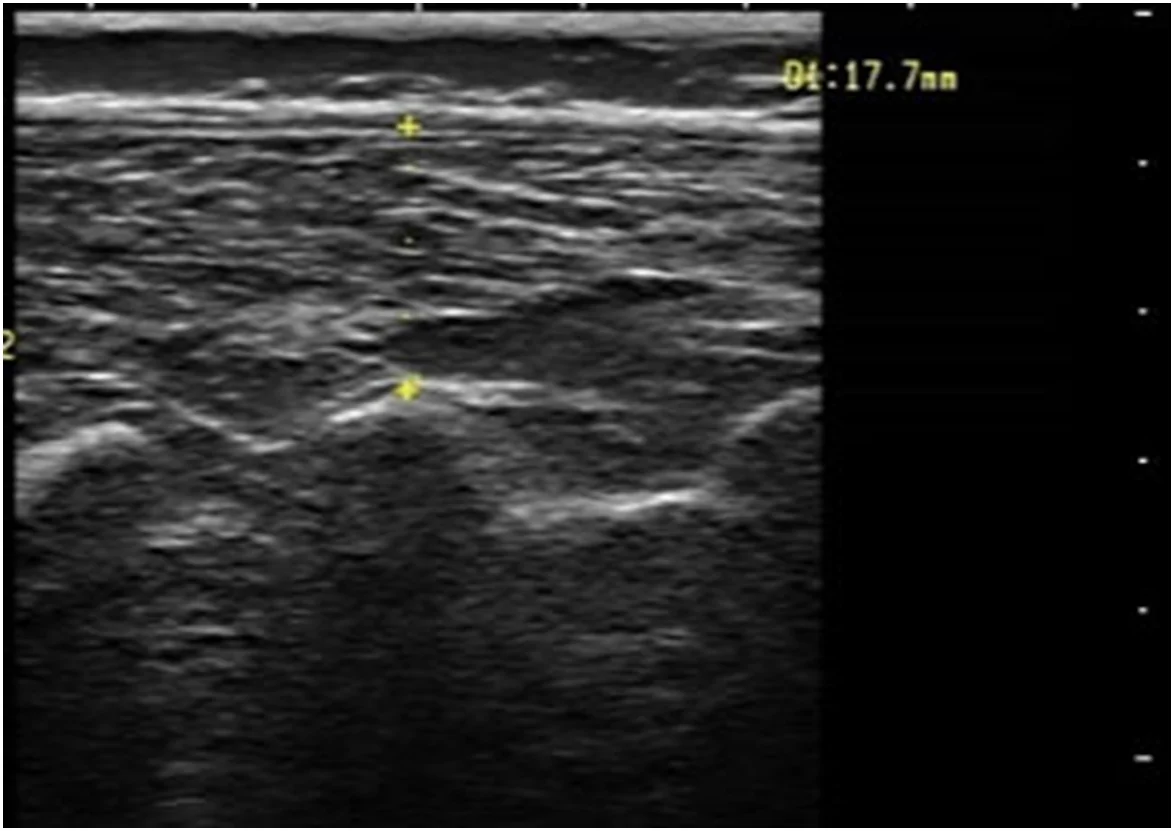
Ultrasound image of the thickness of the Multifidus muscle at rest. Created by the authors. Published under CC BY 4.0.
